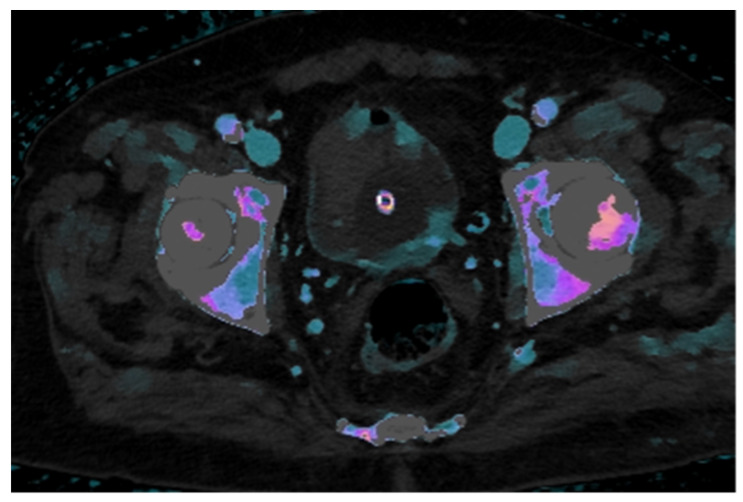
The iodine density map overlaid on venous phase CT images shows increased iodine uptake in the posterolateral left bladder wall and in two foci of the anterior wall, findings indicative of metabolically active neoplastic areas. In contrast, the mass in the right anterolateral wall demonstrates no iodine uptake, consistent with a non-metabolically active lesion, suggestive of thrombus.
